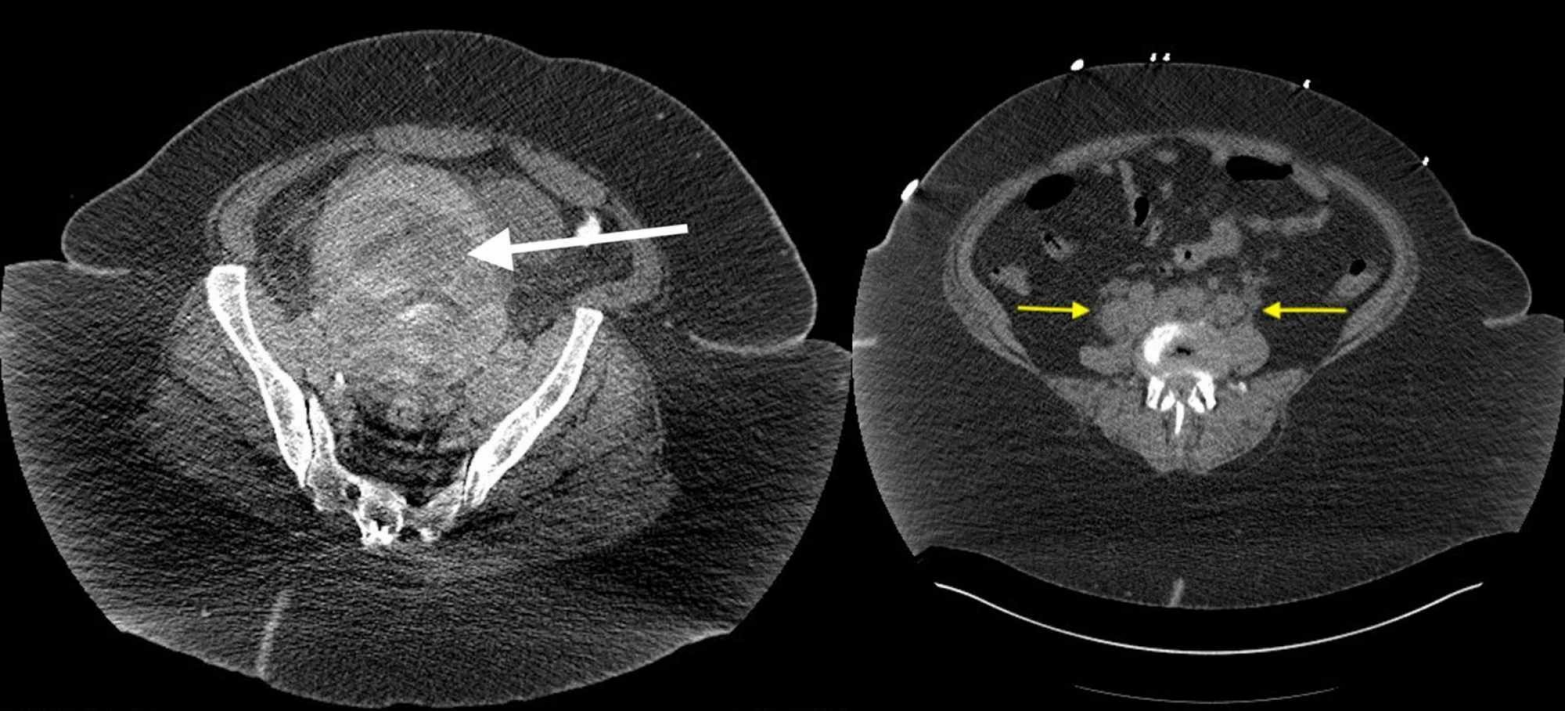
Computed tomography of the abdomen and pelvis showing a large uterine (white arrow) and retroperitoneal lymphadenopathy (yellow arrows)
